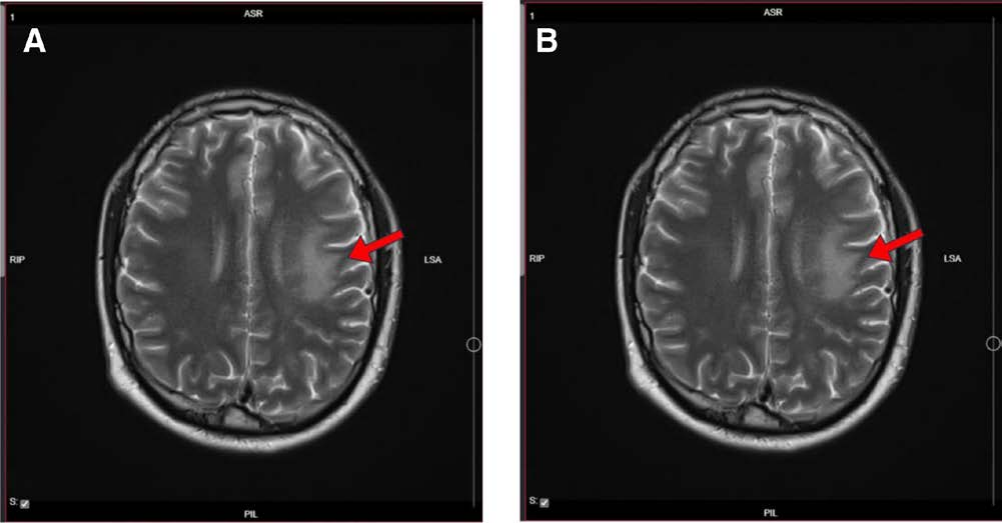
(A) T2-weighted MRI showing hyperintense lesions in the periventricular and subcortical white matter, consistent with areas of active inflammation and demyelination seen in ADEM. The red arrow indicates one of these lesions. (B) FLAIR MRI showing the same hyperintense lesions, one of which is indicated by the red arrow, with better delineation due to the suppression of the CSF background. ADEM = acute disseminated encephalomyelitis, CSF = cerebrospinal fluid, FLAIR = fluid attenuated inversion recovery.
